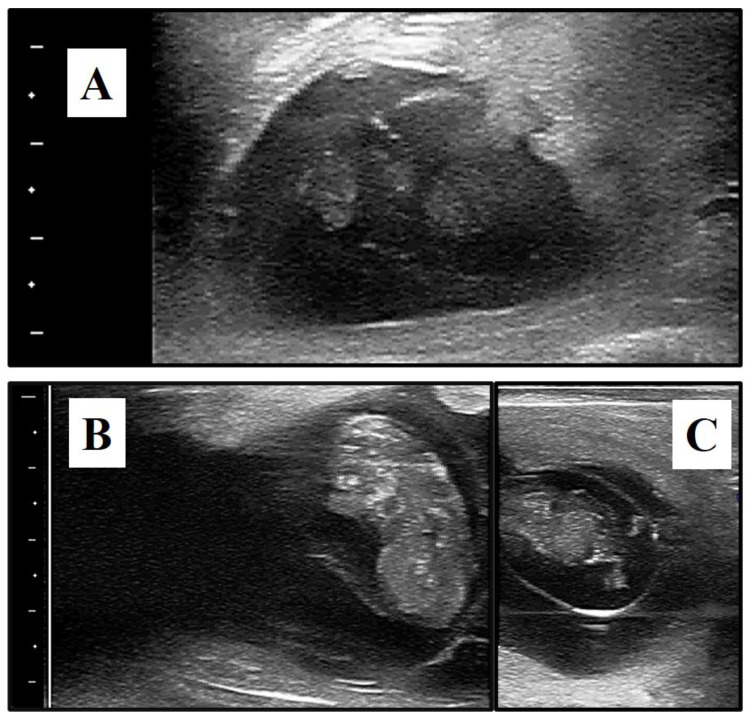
Sonograms showing signs of degeneration in both twins and turbidity of the uterine content 34 days post-AI (A) or one fetus seemingly of normal size and development (B) alongside its partner showing signs of deterioration such as floating hind limbs within the amnion at 52 days post-AI (C). Bar spacing: 10 mm.
